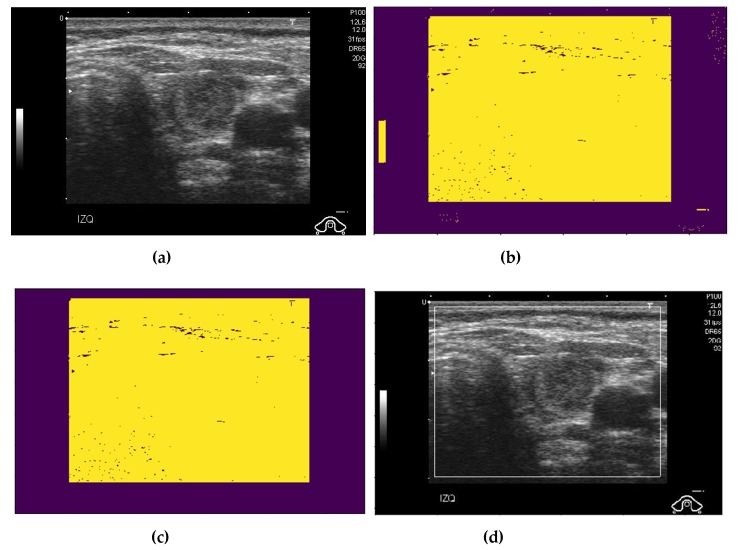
Example result of the thyroid region detection algorithm used in our study: (a) an input ultrasound thyroid image; (b) the binarized image; (c) the thyroid region detection by selecting the largest object; and (d) the final detection results.
